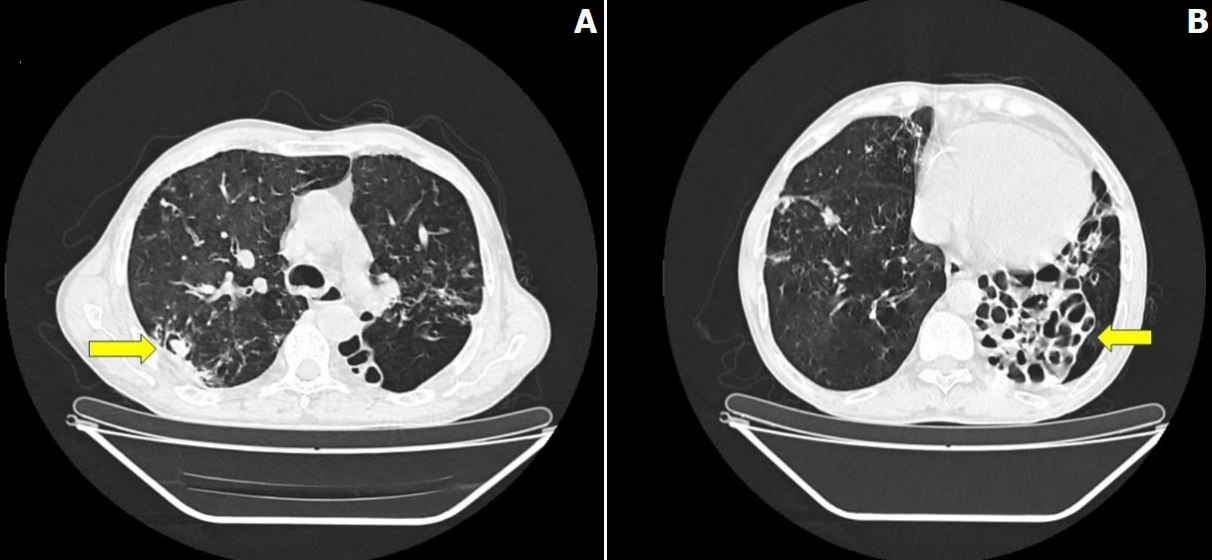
A) CT of the thorax with yellow arrow showing cavity with hypodense content depicting air crescent sign; B) CT of the thorax with yellow arrow showing cystic bronchiectasis in the left lower lobe
